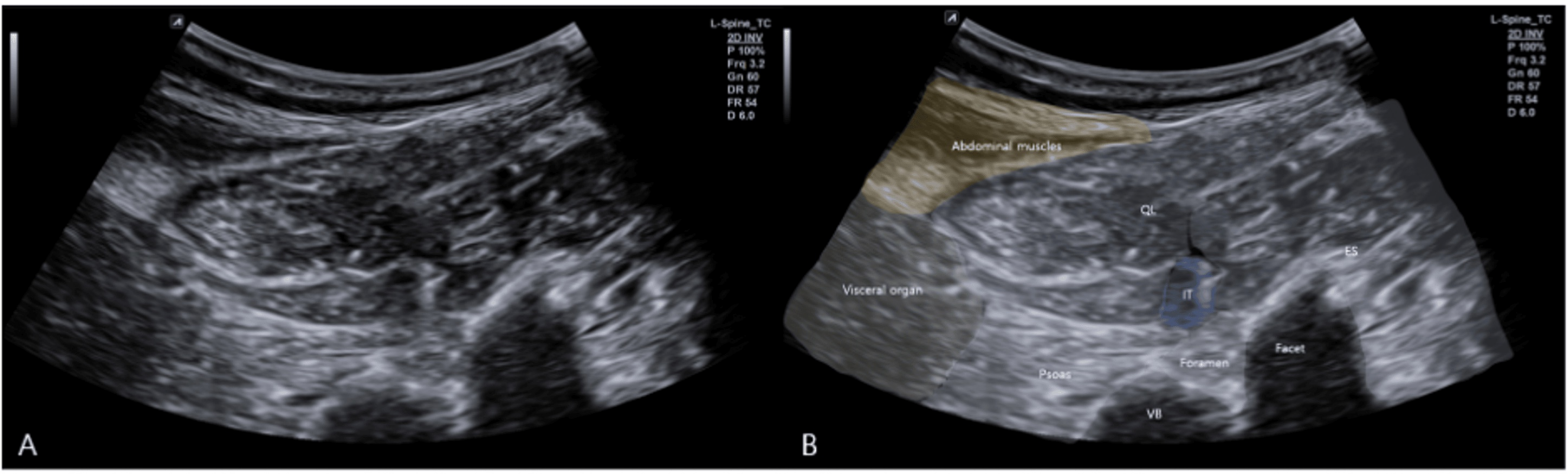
Right lateral decubitus ultrasound setup for intertransversarii assessment Right lateral decubitus setup and lateral acoustic window
